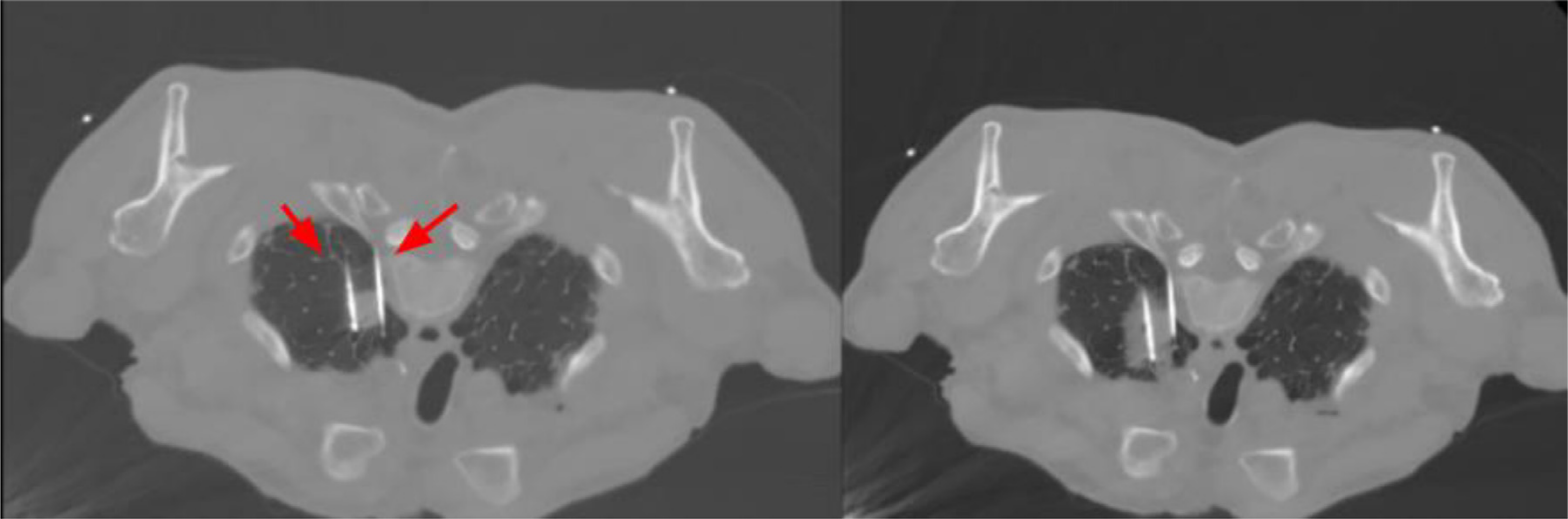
Thoracic CT of the second patient during the cryoablation (mediastinal window). In this patient, 2 needles (red arrows) are used in order to create an ablation zone with safety margins due to its size. The CT images are presented in interventional orientation, where posterior anatomical structures appear at the top of the image.
